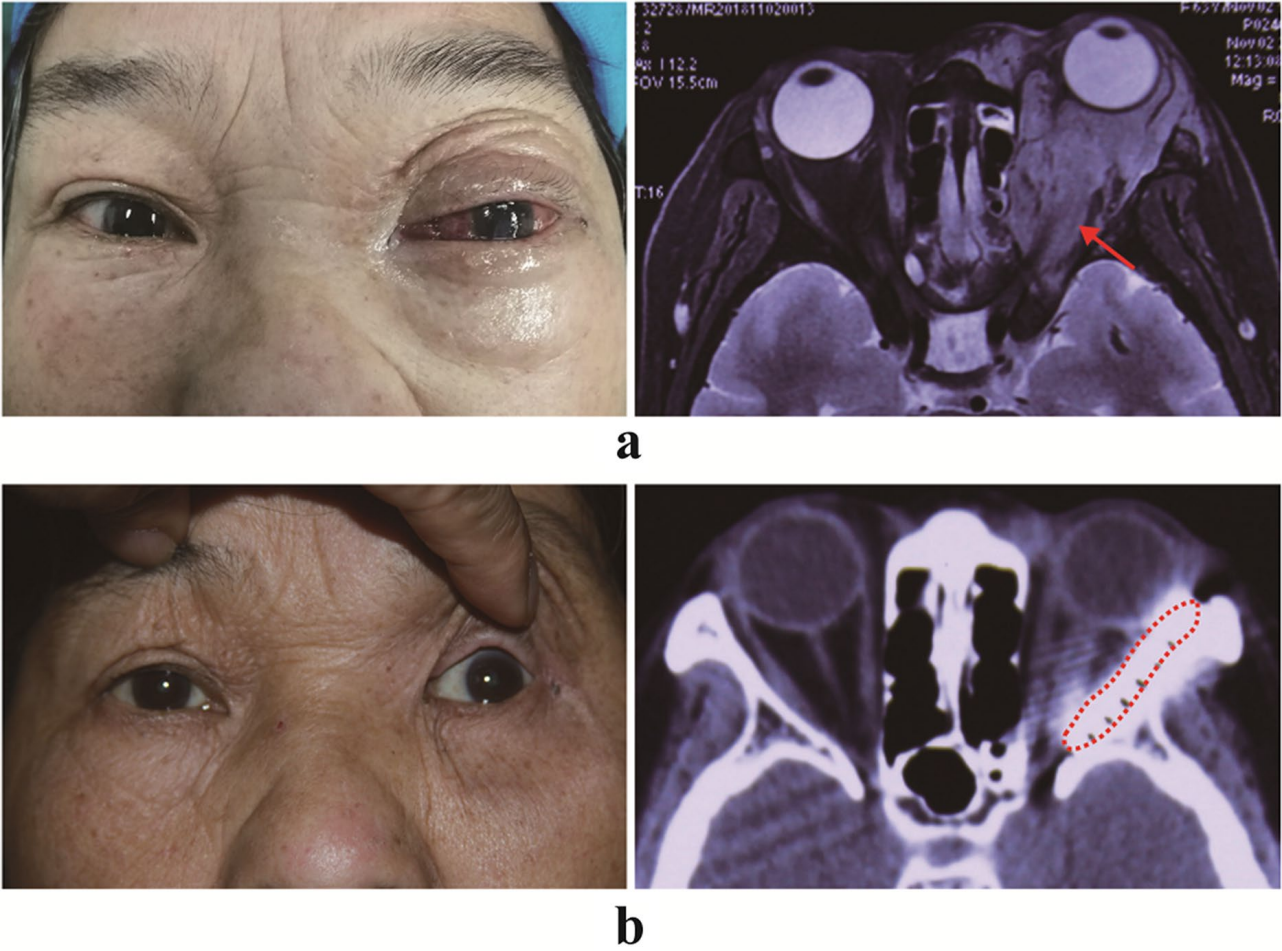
Pre- and postoperative images of patient C. a Preoperative images of patient C The photograph of the patient's appearance shows the left eyeball protruding and a severe corneal ulcer. A CT scan shows a large soft tissue mass in the left whole orbital region (red arrow). b Twenty months after brachytherapy. The photograph of the patient's appearance shows no proptosis and no periocular radiation dermatitis. The left cornea became clean, and the patient’s vision significantly improved. The CT scan shows that the I-125 seeds under the orbital periosteum are stable (red dashed box)
